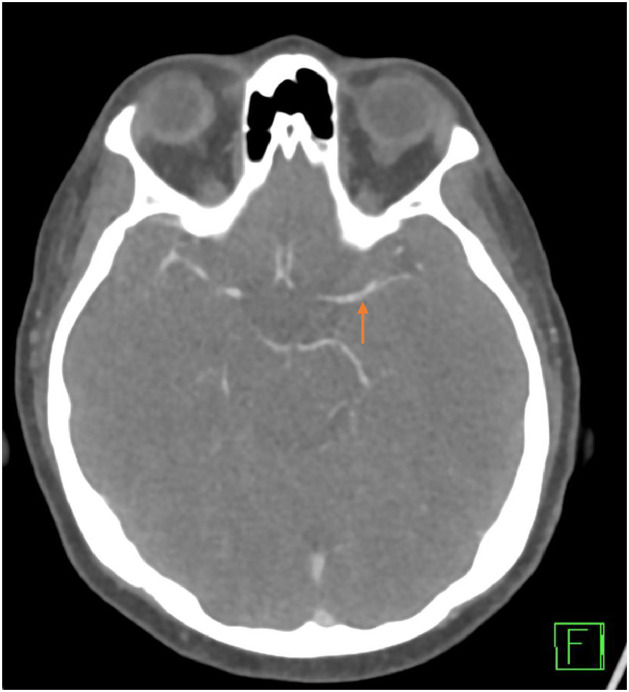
Single selected axial CTA shows the middle cerebral artery not occluded by thrombus or stenosis.
